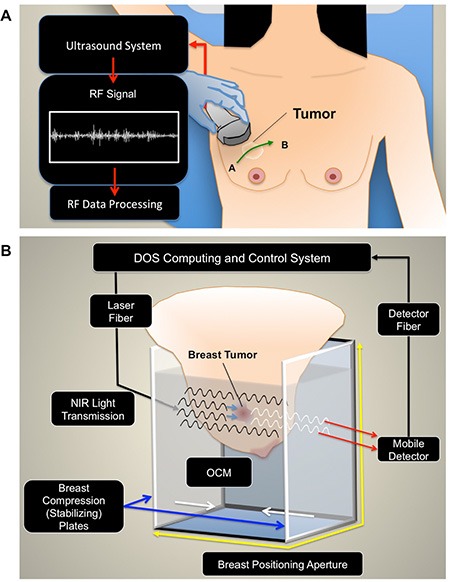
Experimental setup (A) QUS imaging. For QUS, patients were positioned supine for ultrasound imaging. A panoramic scan was acquired over the affected breast, which included the entire volume of the tumor, and also normal breast tissue (points A and B). (B) Diffuse Optical Spectroscopy Imaging. Immediately after sonography, patients were transferred onto a diffuse optical tomography device. The breast was positioned into an imaging aperture and compressed by stabilizing plates to limit motion, and maintain similar breast thickness throughout the scan series. Optical compensation fluid was added to the imaging aperture to improve light transmission between the varying surfaces.
